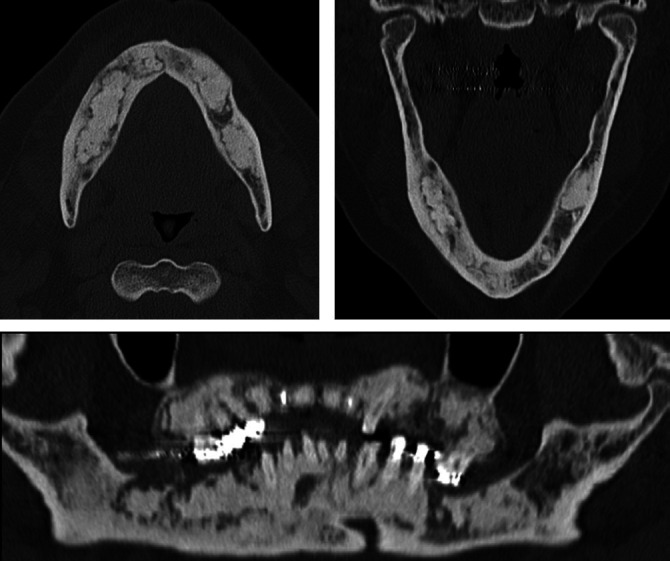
CT scans of a 50-year-old female patient, showing multiple
